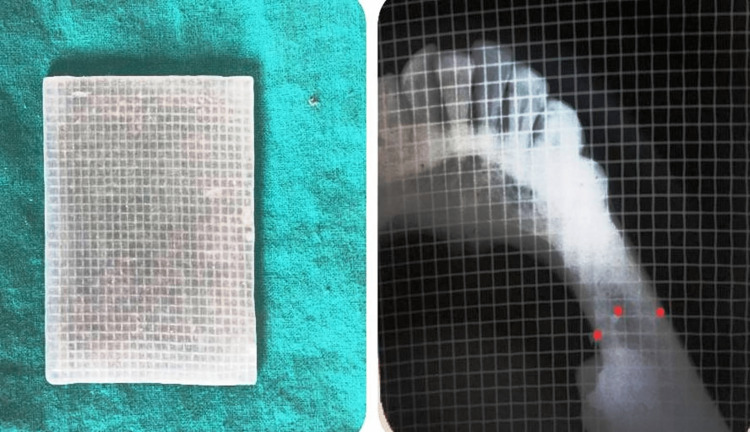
The image on the right shows a customized radiographic mesh, and the image on the left shows alveolar ridge measurement by intraoral periapical radiograph in the occlusal projection (red dots showing marking).
